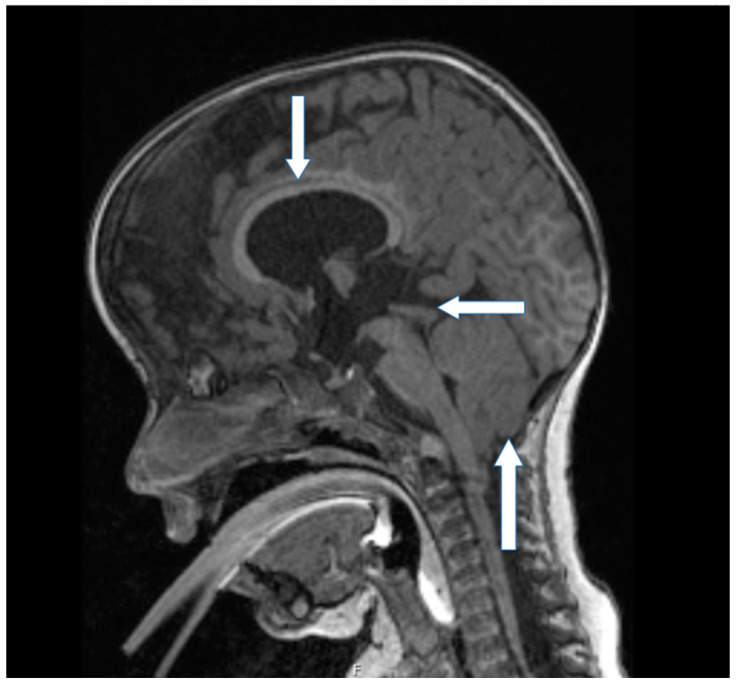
Non-enhanced sagittal MRI T1-weighted scan revealed a Chiari malformation type 2 (up arrow), hypoplasia of the corpus callosum (down arrow), and dysgenesis of the lamina tecti (left arrow).
